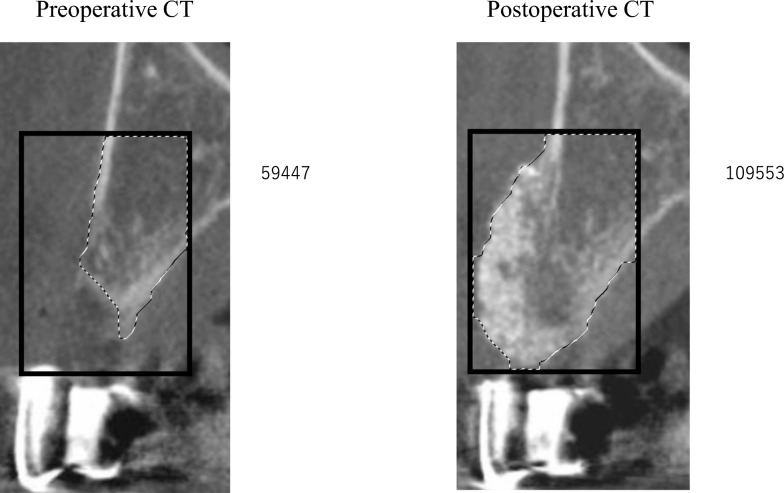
Comparative analyses of preoperative and postoperative CT images of the grafted site. Pre- and post-operative sites were aligned as closely as possible using radiopaque markers, and crestal bone width and grafted area outlined with dotted lines were measured using image analysis software
